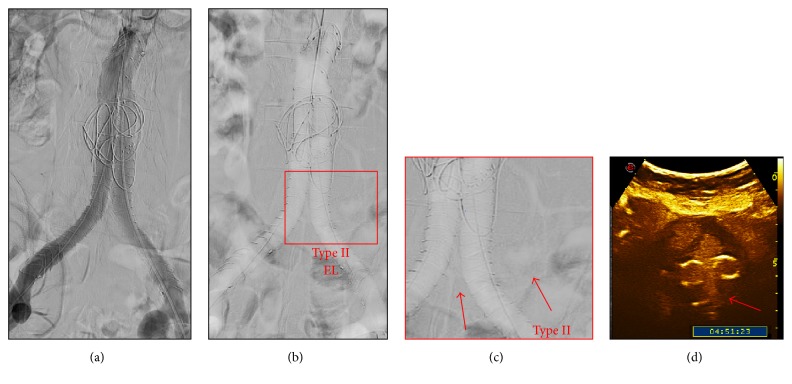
(a) Iodinated contrast media completion angiography that shows the good positioning of the infrarenal fixation endograft and the absence of endoleaks. (b) Carbon dioxide completion angiography that shows the good positioning of the infrarenal fixation endograft and the presence of ELII. (c) Magnification of ELII from sacral artery (red arrows indicates ELII coming from sacral artery). (d) Contrast-enhanced ultrasound that shows the presence of ELII with the inflow from sacral artery (red arrow).
